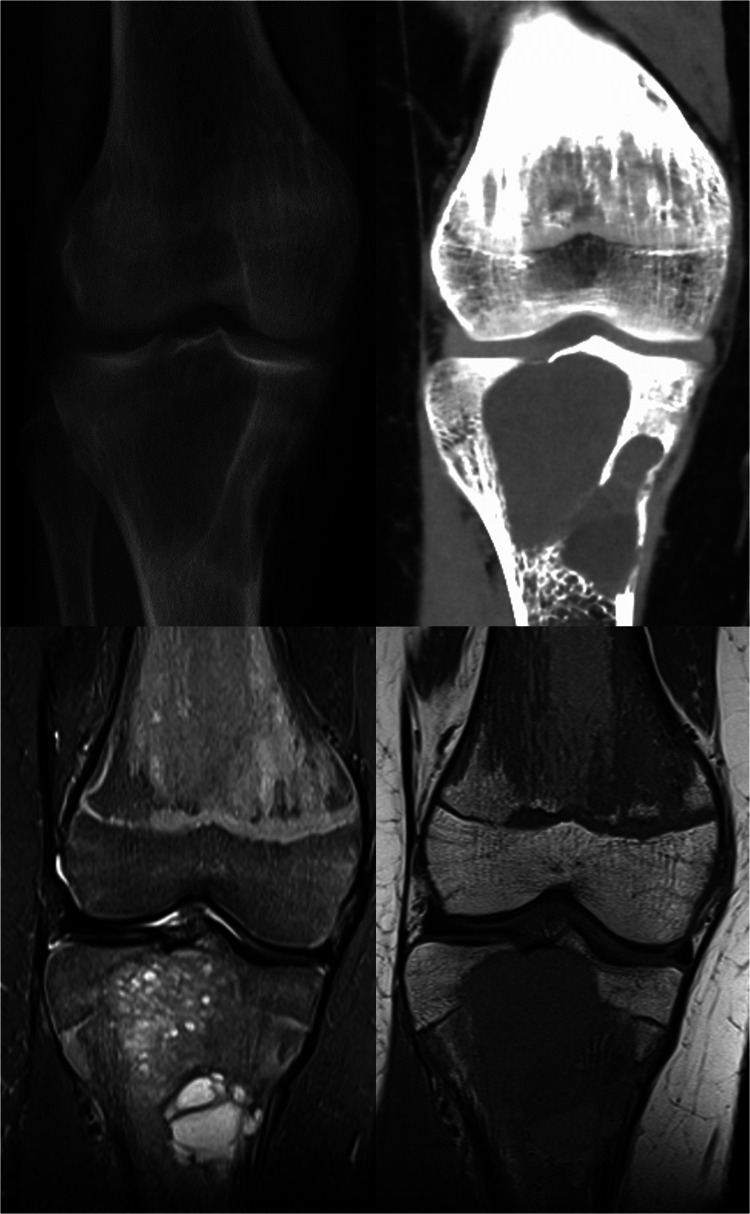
Sixteen-year-old female with Brown tumour. Coronal average intensity projection radiograph-like (top left) and CT reconstruction (top right) images showing lytic lesions within the proximal tibia of varying attenuation. Expansion related to the inferior lesion results in endosteal scalloping and deficiency of the medial cortex. Coronal STIR (bottom left) and T1-weighted (bottom right) images show complex corresponding signal characteristics with sub-physeal resorption best appreciated related to the distal femoral physis
